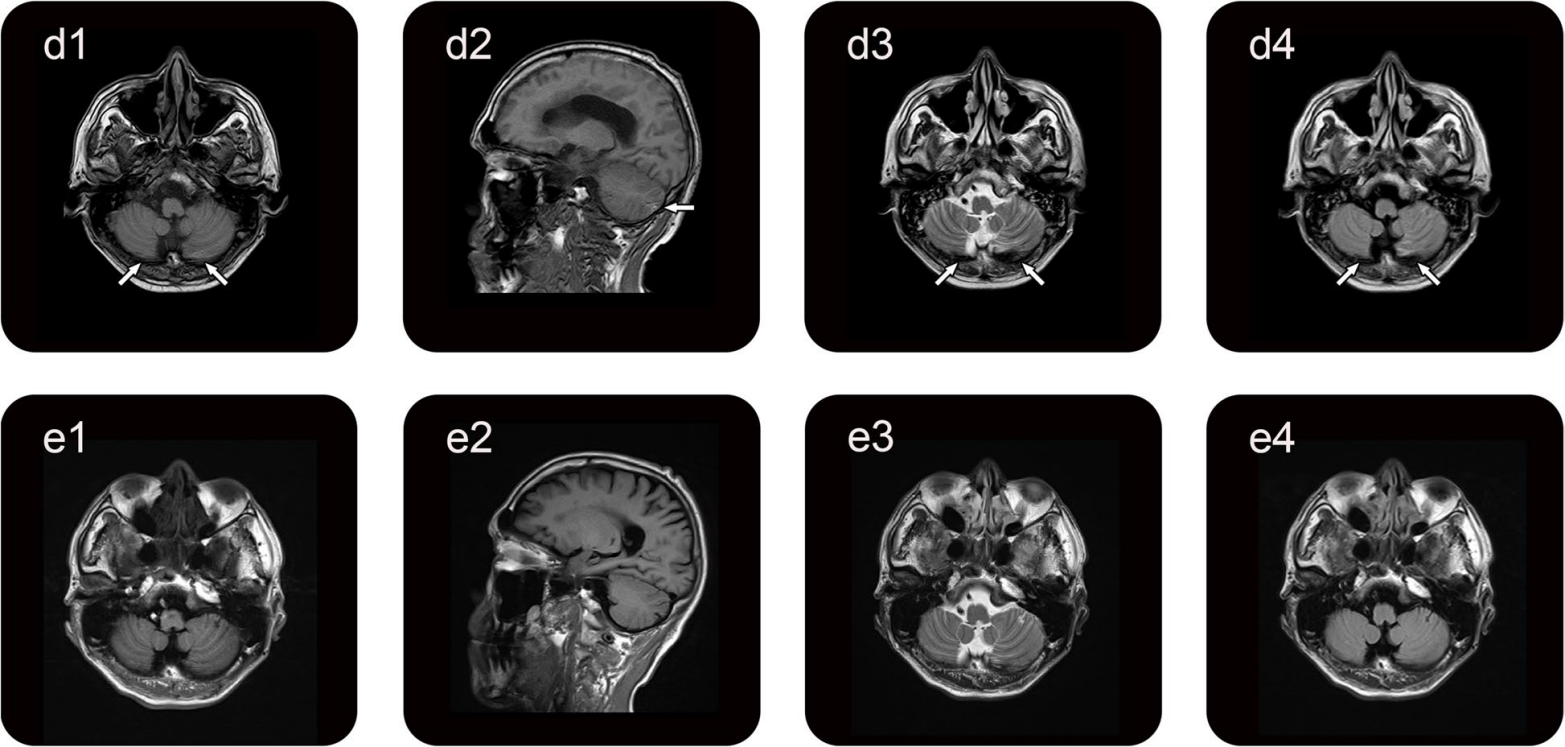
The follow-up brain MRI scans after discharge. Brain MRI, 1 month later, showed a little streaky T1WI hyperintensity (d1, d2; arrows) with T2WI and T2 FLAIR hypointensity (d3, d4; arrows). A follow-up brain MRI, 1 year later, showed no abnormal findings (e1-e4)
